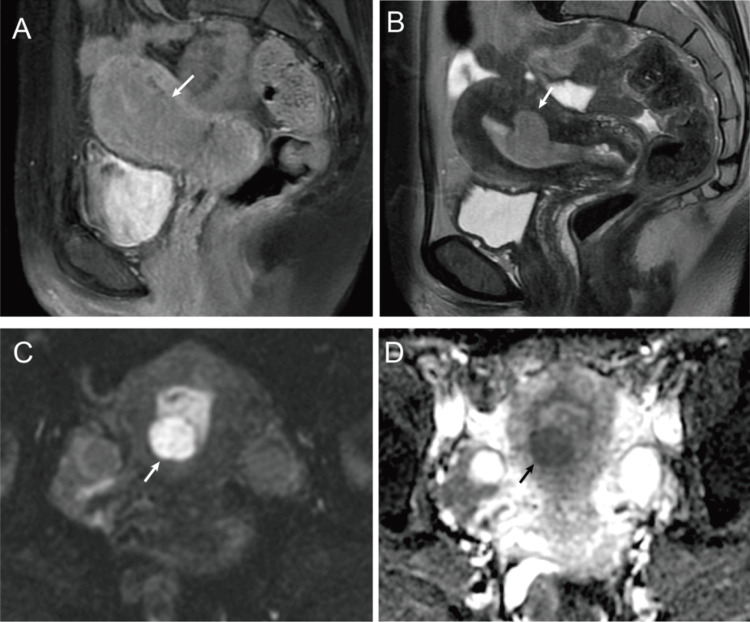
Forty-five-year-old woman with LGESS (case 5) A mass was located in the uterine posterior wall (arrow), showing isointensity on T1WI (A) and hyperintensity on T2WI (B). This mass showed high signal intensity upon diffusion-weighted MRI (C) with a low ADC value (arrows) (D). LGESS: low-grade endometrial stromal sarcoma; T1WI: T1-weighted imaging; T2WI: T2-weighted imaging; MRI: magnetic resonance imaging; ADC: apparent diffusion coefficient
